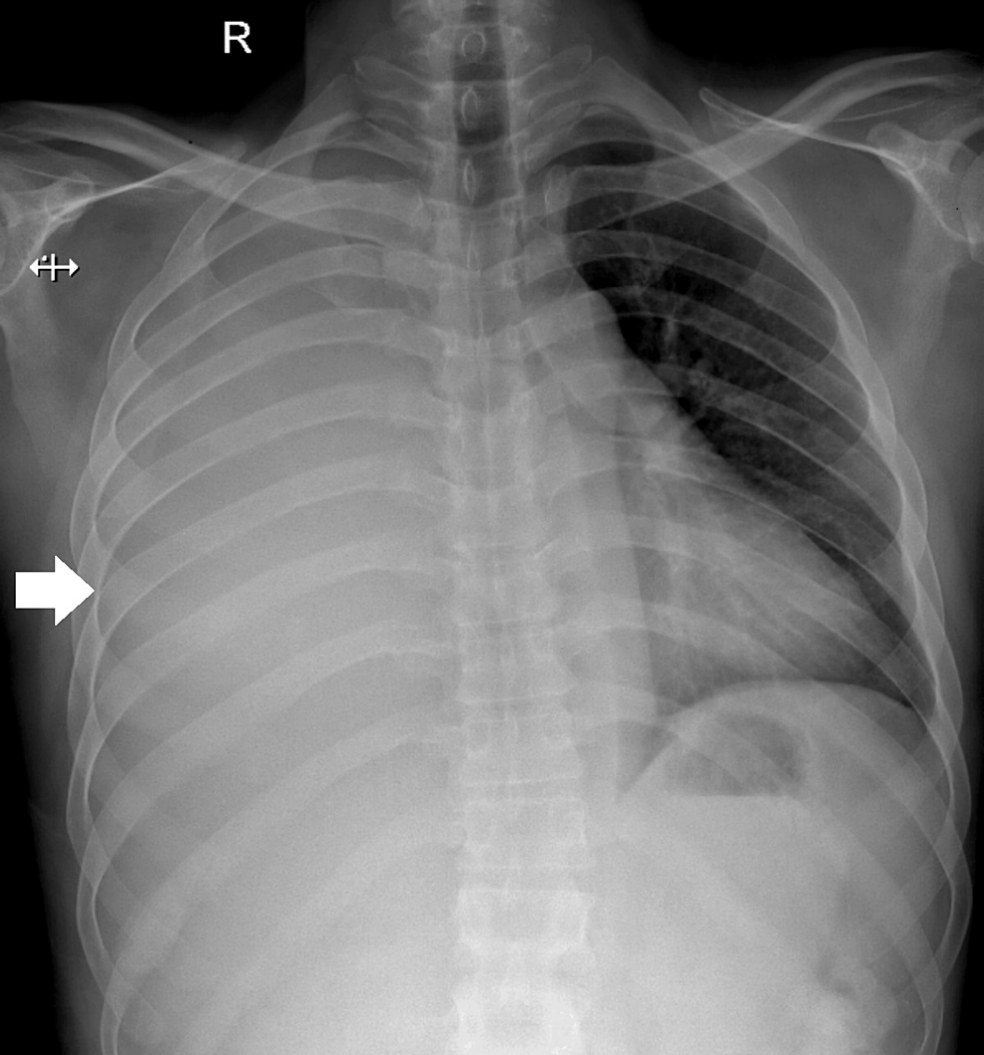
Chest radiograph with significant right pleural effusion (white arrow) with mild mediastinum shift to the left
